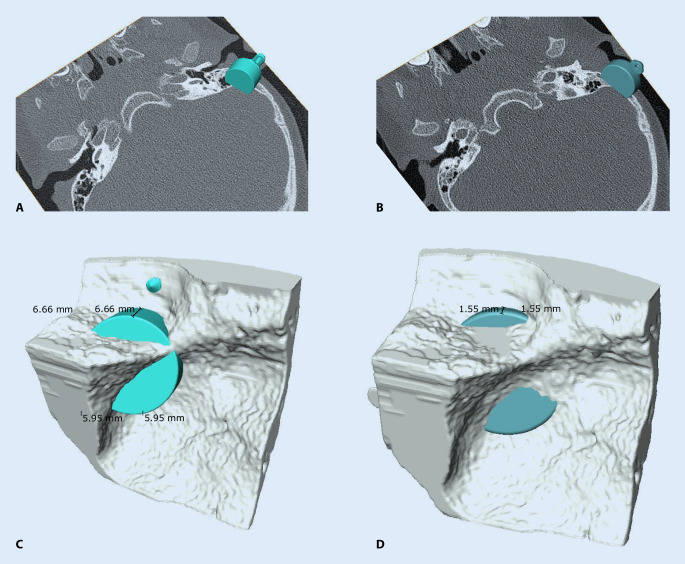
Preoperative plan for the implantation of an active, transcutaneous bone conduction hearing system in a 4.5-year-old patient with atresia and complete conductive loss (according to Plontke et al. 2014 [17]). The bone conduction-floating mass transducers required intracranial penetrations of: (A, B) 6–7 mm for the BCI 601 and (C, D) <2 mm for the BCI 602. Due to the expected skull growth [25], a computed tomography scan and planning were not repeated before surgery at the age of 5.5 years. Intraoperatively, the dura and sigmoid sinus were exposed, but not impressed. This patient is also shown in Fig. 4
